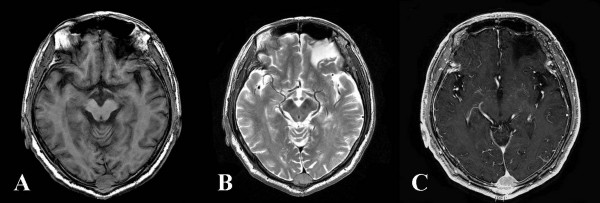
Magnetic resonance imaging (MRI) of the skull metastasis from hepatocelluar carcinoma. A) T1-weighted MRI and B) T2-weighted MRI demonstrating a homogeneous, well-defined, and iso-signal intensity mass in the occipital midline. C) Gadolinium enhanced T1-weighted MRI images showing a strong enhancement of the tumor.
